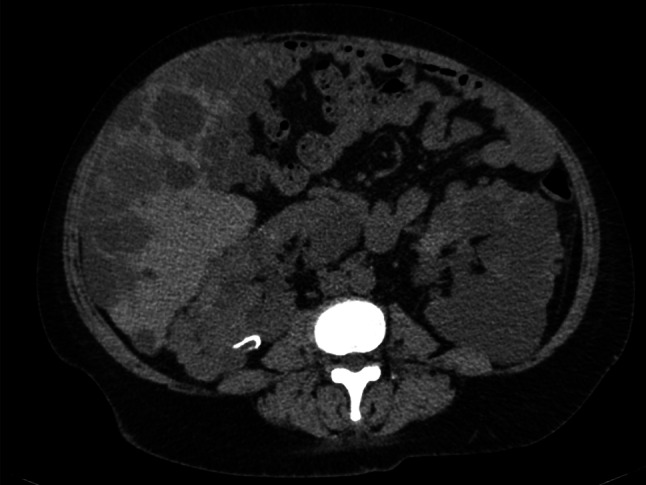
Computed Tomographic appearance of both kidneys. Non-contrast CT scan obtained at the time of admission reveals multiple cysts in both kidneys
